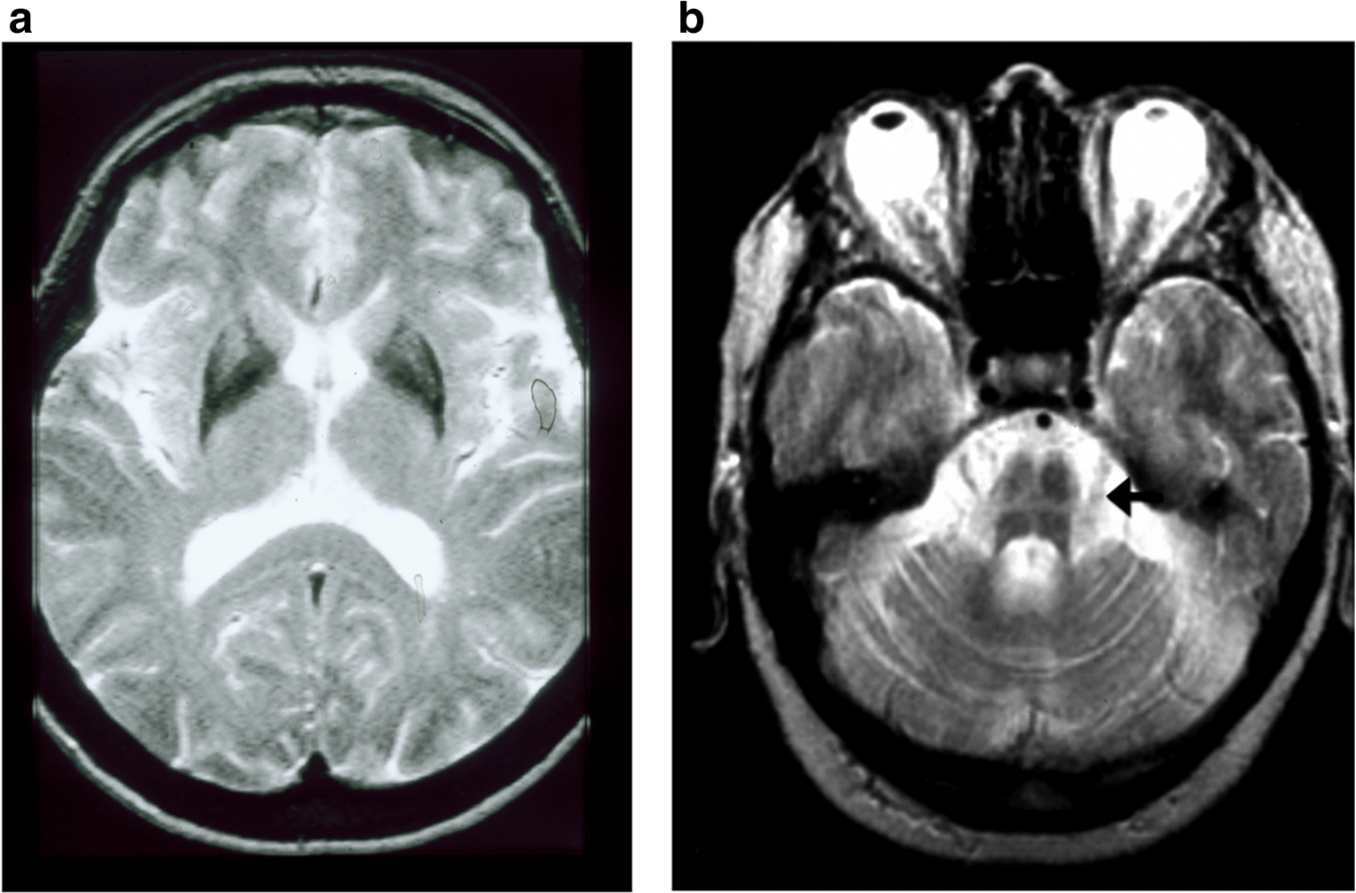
(a) T2-weighted MRI showing a low lateral putamen signal at the same level as the pallidum in MSA. (b) T2-weighted MRI showing the pontine hot cross bun sign in MSA
